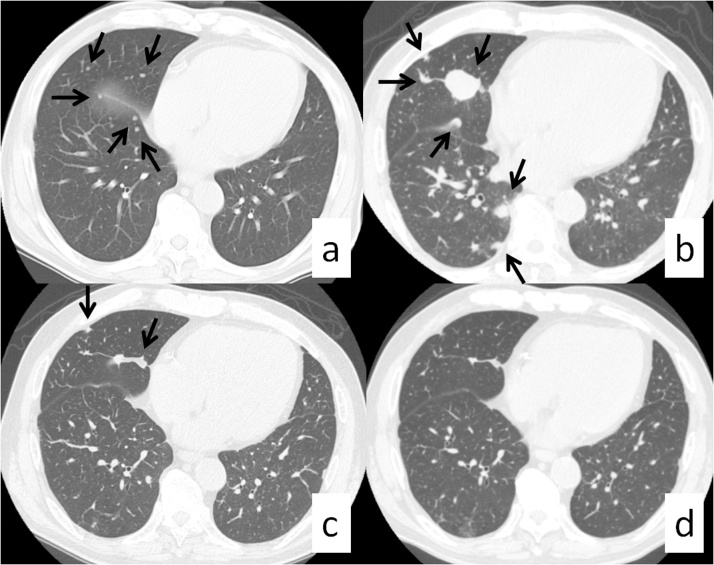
(a) CT chest revealed innumerable lung nodules concerning for metastatic disease. (b)The follow-up chest CT scan revealed progression of the lung tumor 13 months after the first presentation. (c)The follow-up chest CT scan revealed spontaneous regression of the lung tumor 18 months after the first presentation. (d) Almost complete disappearance of the lung metastasis 41 months after the first presentation (arrow).
